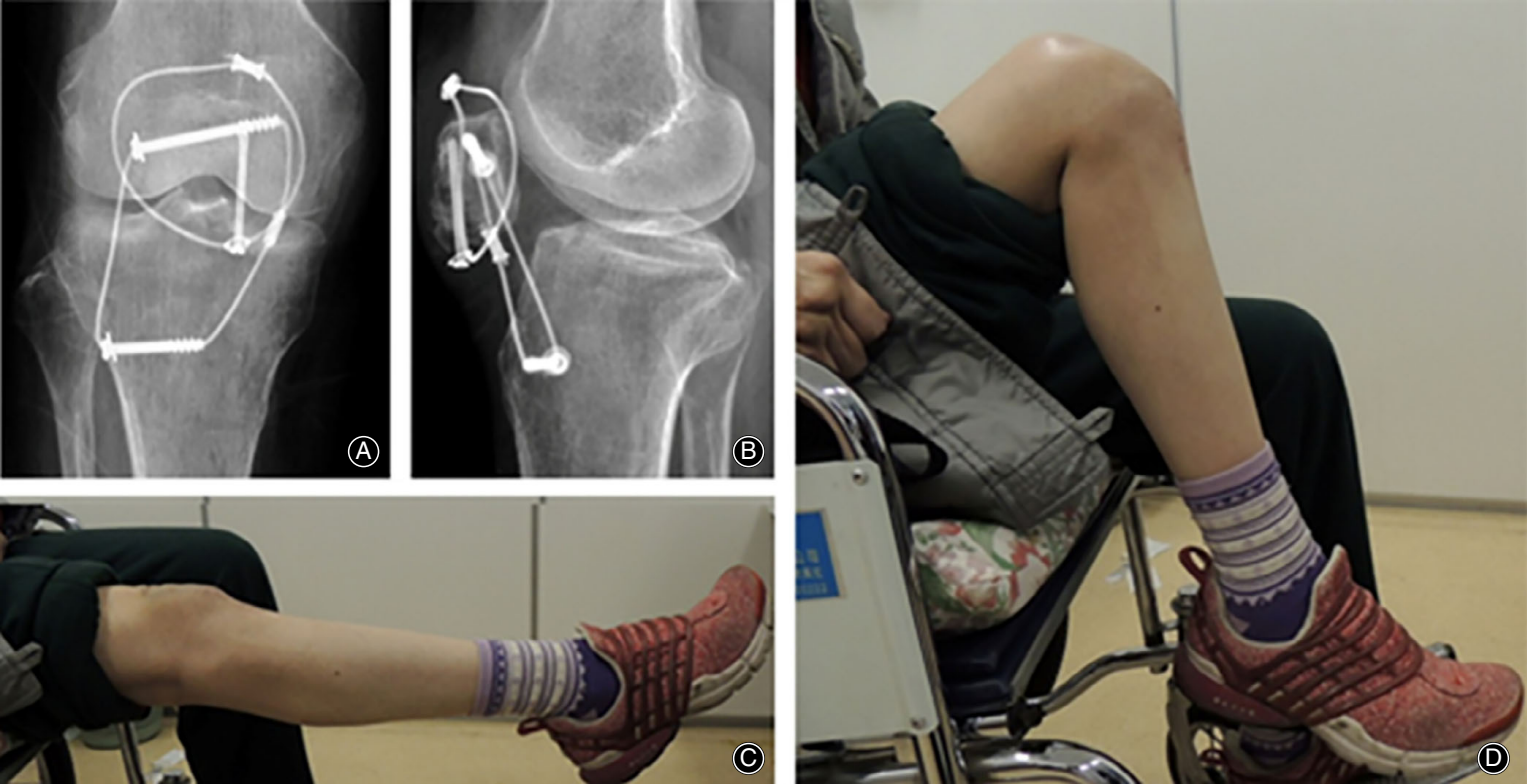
(A) and (B) A total of 8 weeks postoperatively, X‐ray radiography showed good internal fixation and fracture healing. (C, D) Knee activity was 10° to 100° 8 weeks postoperatively, without weight‐bearing walking.
